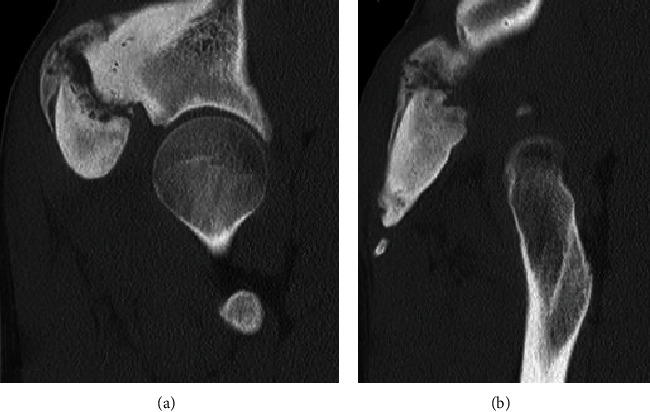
(a) Coronal CT image demonstrating the right AIIS and the large amount of heterotopic ossification. (b) Another sequence from the coronal CT imaging demonstrating the extent of bone growth.
